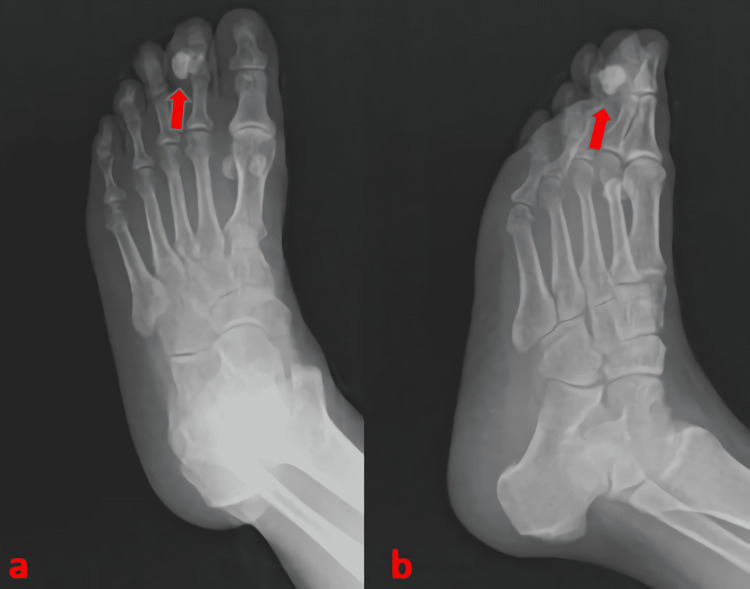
Preoperative X-ray images.
